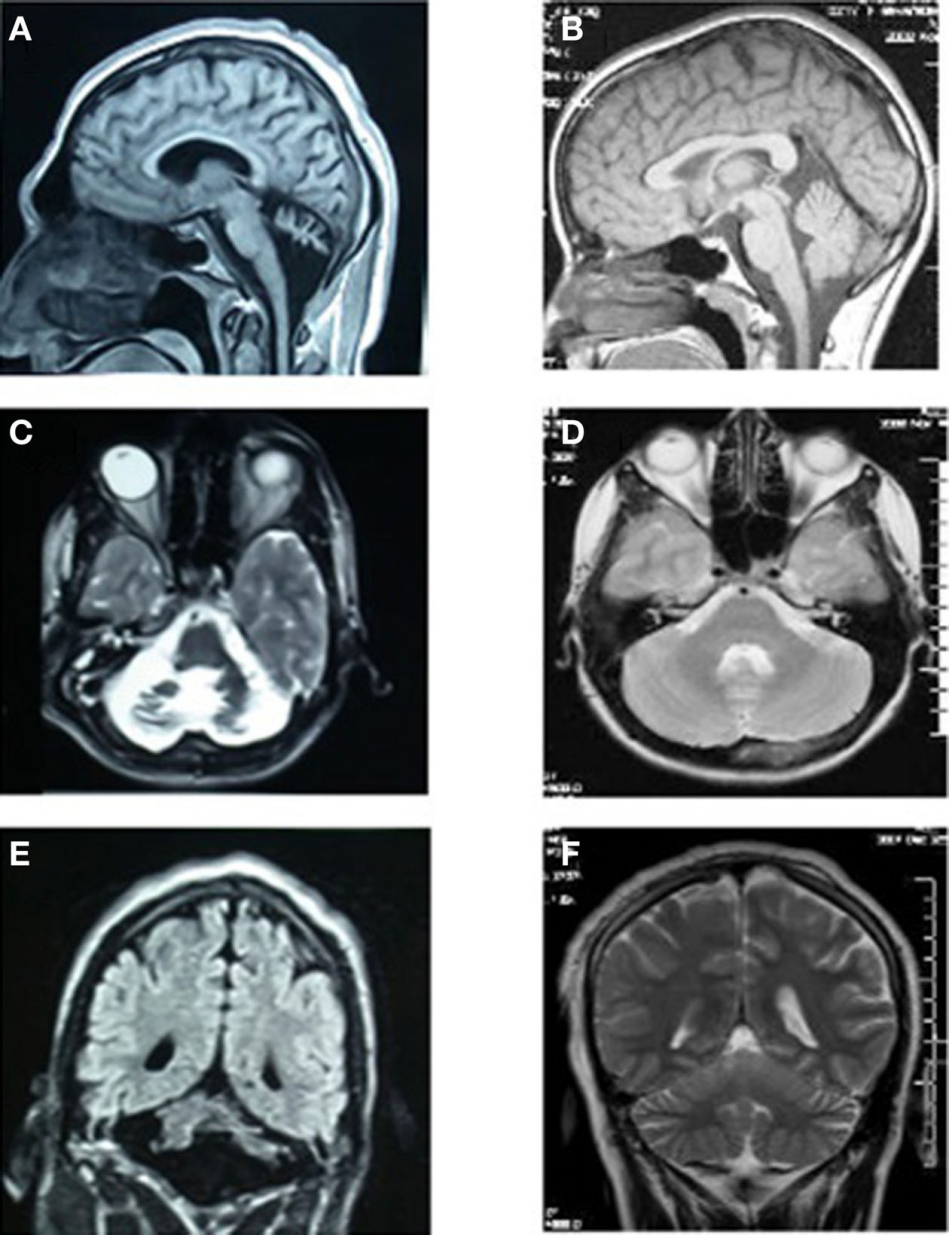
MRI scans of the patient IV-16 (left) and the unaffected relative (right). (A,B): midsagittal; (C,D): axial; (E,F): coronal sections.
